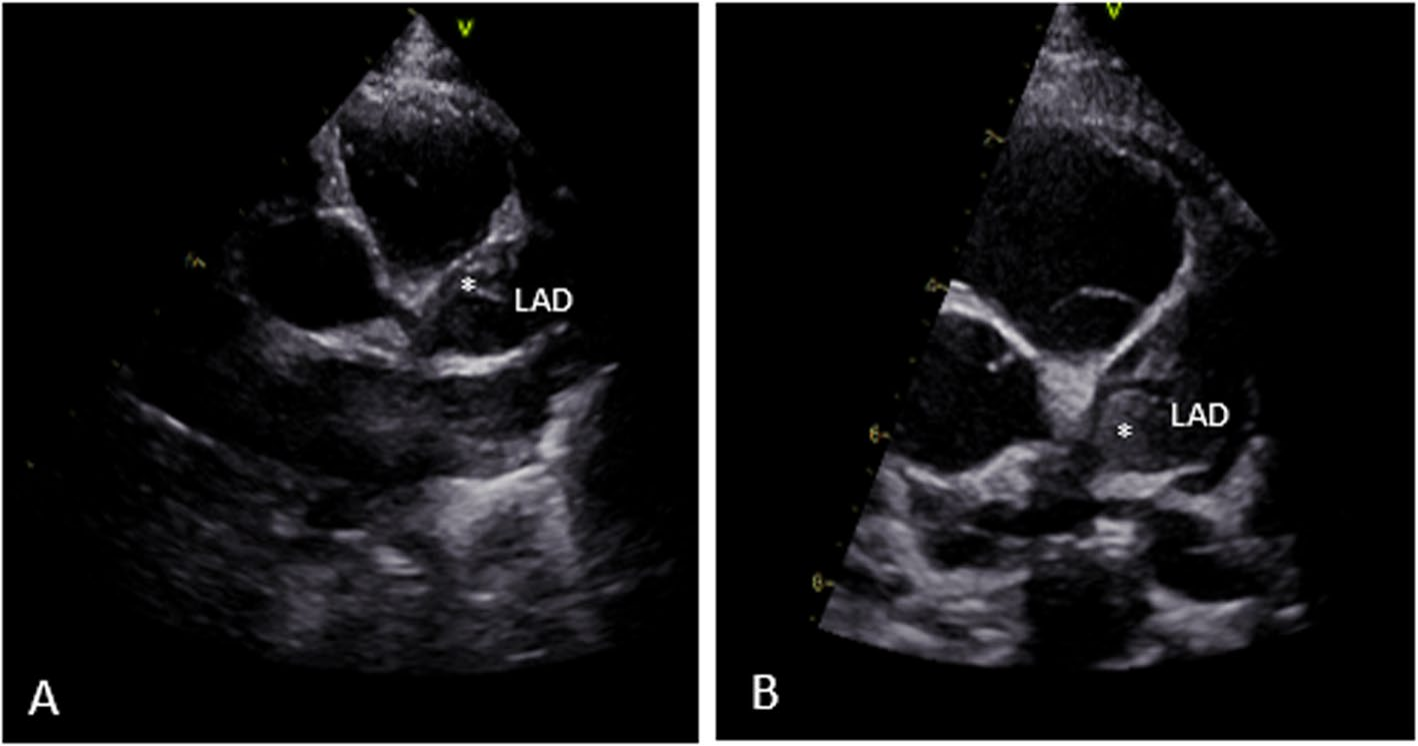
Thrombus growth depicted by echocardiography. A Thrombus (*) in LAD. B Thrombus (*) growth in the course of 4 days in LAD
